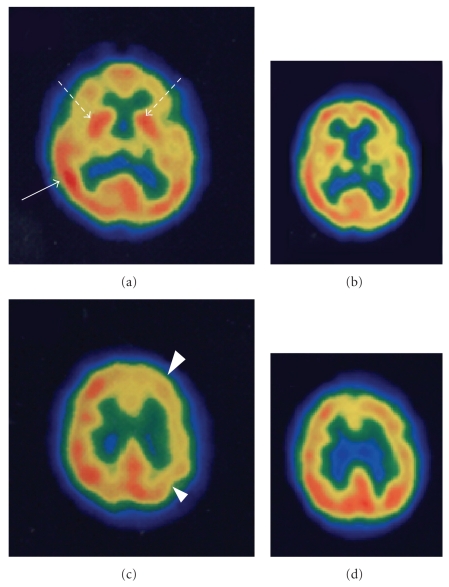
99^m^-HMPAO-SPECT images acquired during a seizure and 10 days later. (a) and (c) show relative hyperperfusion in the right parietal lobe (arrow) and both thalami (dotted arrows) and relative hypoperfusion in the left prefrontal and parietal lobes (arrow heads) during a seizure. (b) and (d) are the same axial slices as (a) and (c), respectively, obtained 10 days after the final seizure. In (b) and (d), relative hyperperfusion and hypoperfusion are not noted, as bilateral symmetrical cerebral perfusion has been normalized.
